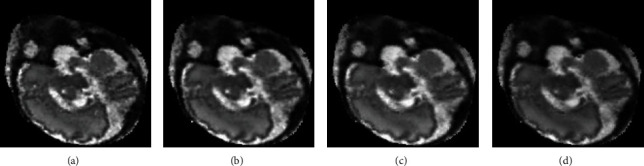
Reconstructed results of the MRI data with different kernel window sizes: (a) *k*_*s*_ = 3, (b) *k*_s_ = 5, (c) *k*_s_ = 7, and (d) *k*_s_ = 9.
